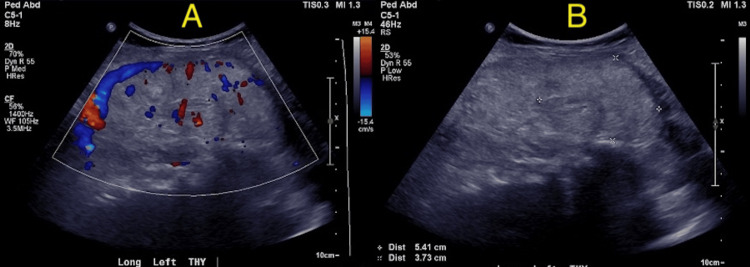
Color Doppler image of the left thyroid nodule in (1A) demonstrates internal vascularity of the left thyroid nodule, and image (1B) shows a solid, isoechoic, wider-than-tall lesion with smooth margins and no echogenic foci measuring 5.4 x 3.7 x 2.7 cm within the inferior pole of the thyroid gland. This lesion is classified as a thyroid imaging reporting and data system 3 (TI-RADS 3) lesion.
